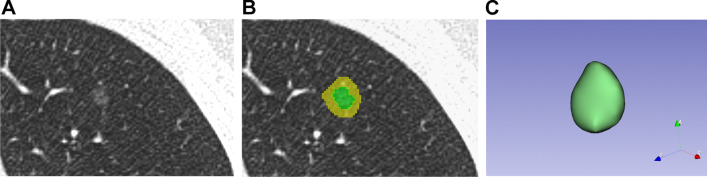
The segmentation of the regions of interest. A Computerized tomography (CT) image of a ground-glass opacity nodule pathologically confirmed as atypical adenomatous hyperplasia (AAH). B The segmentation of the nodule. Green area indicates the nodule region and yellow area indicates the peri-nodule region. C The constructed 3D model of the nodule in 3D slicer software
